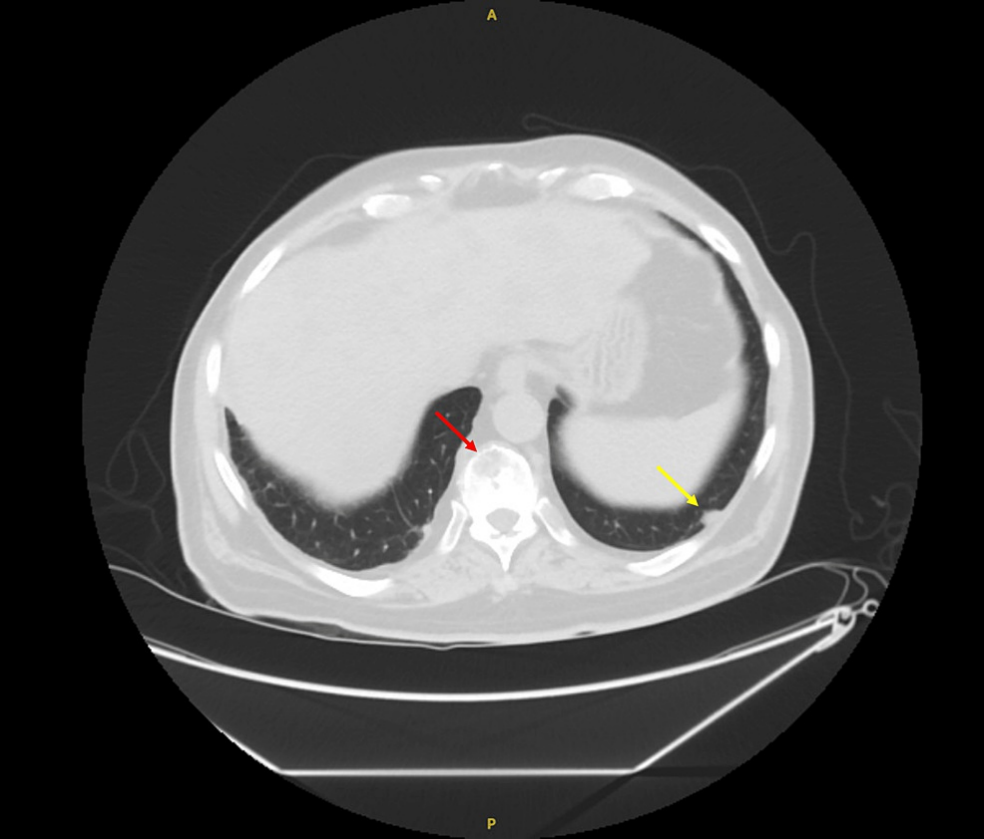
The CT scan shows one of the multiple lung lesions (yellow arrow) and one of the multiple lytic bony lesions (red arrow).
